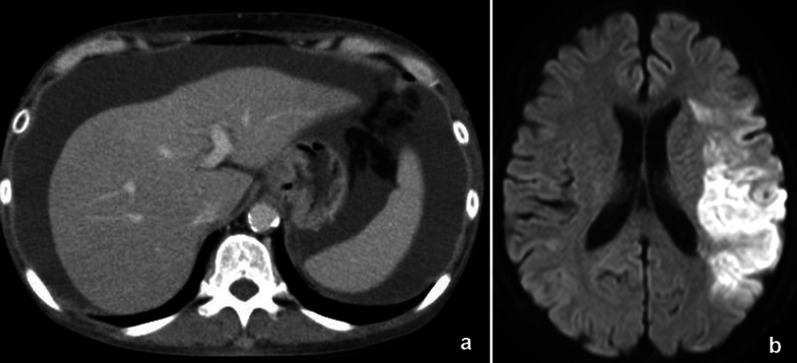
Imaging findings at onset of brain infarction. a. Enhanced computed tomography image showing abundant ascites; b left brain infarction presented by magnetic resonance imaging
